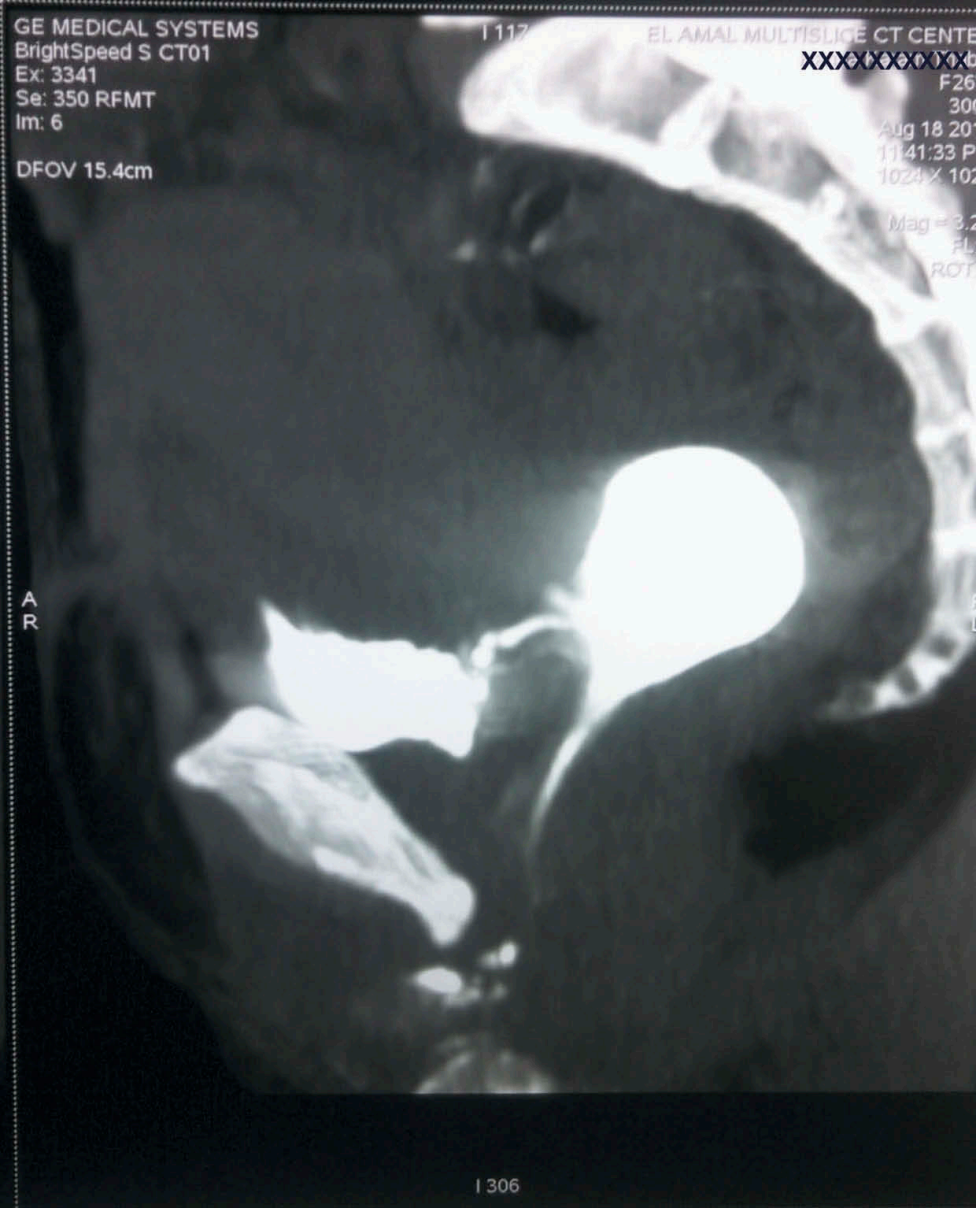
Lateral CT cystogram with contrast demonstrating VVF.
